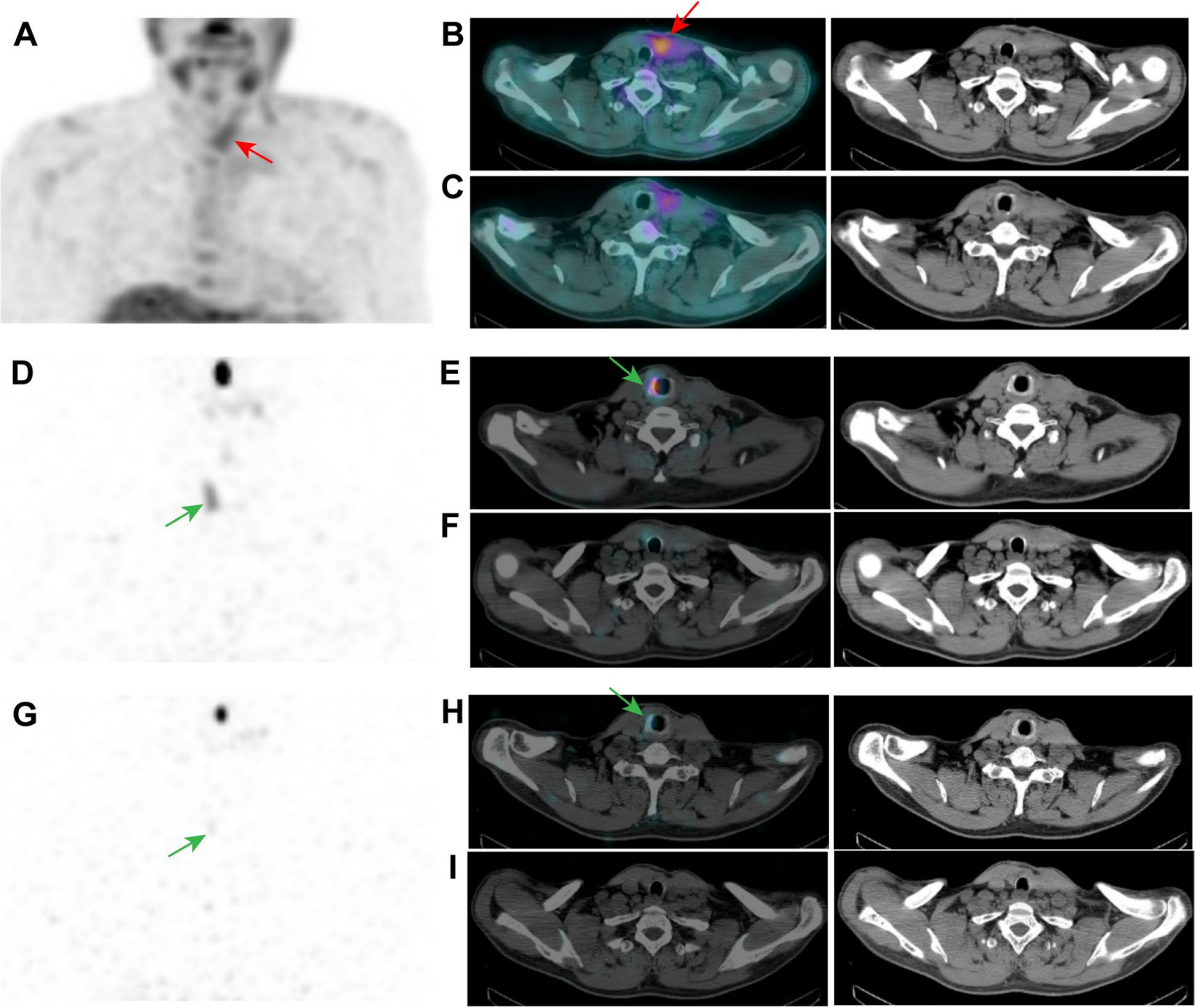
^99m^Tc-3PRGD_2_ SPECT/CT detected local recurrence in a 67-year-old male PTC patient with initial TSH-stimulated Tg 211.5 ng/ml. ^99m^Tc-3PRGD_2_ SPECT/CT before RAI showed a focal uptake in the left thyroid bed in planar image (A) and transaxial fused SPECT/CT (B), which was seen a soft-tissue mass on CT image. ^131^I post therapy WBS found a slight radioiodine uptake in planar image (D) and transaxial fused SPECT/CT (E) in the right thyroid bed. The lesion in the left thyroid bed was negative on ^131^I WBS (F). ^99m^Tc-3PRGD_2_ SPECT/CT showed no ^99m^Tc-3PRGD_2_ uptake in the right thyroid bed (C). Four months after initial RAI, the serum TSH-stimulated Tg of the patient elevated to 734 ng/ml. The neck ultrasound examination also detected local recurrence in the left thyroid bed. The patient received another RAI, and the post-therapeutic WBS showed only a vague uptake of radioiodine in the right thyroid bed (G, H). The lesion in the left thyroid bed was still negative on secondary ^131^I WBS (I)
